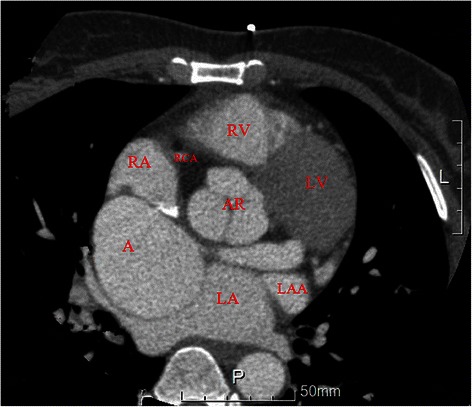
CTA coronary angiography showed the relation between aneurysm and left main trunk. Abbreviations LV: Left ventricle; LA: Left Atrium; RV: Right ventricle; RA: Right Atrium; A: Aneurysm; RCA: Right Coronary artery; LAA: Left Atrium Appendix; AR: Aortic Root
